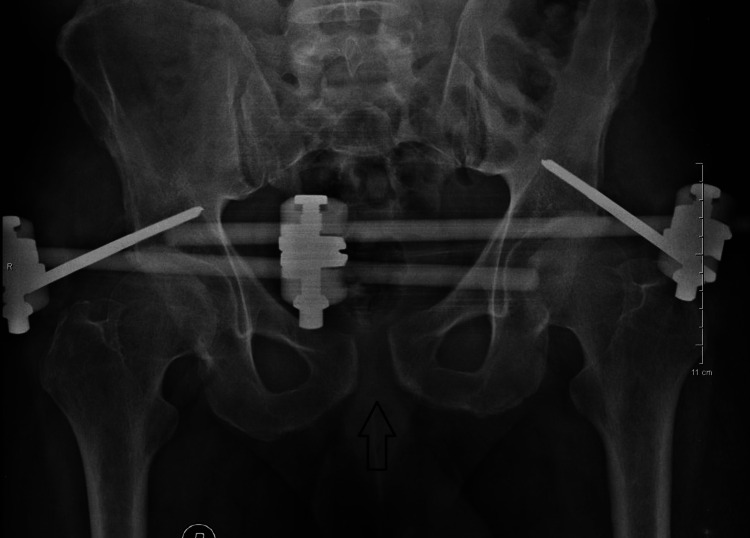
Anteroposterior X-ray after light widening of the pubic symphysis (seven weeks postoperatively).
